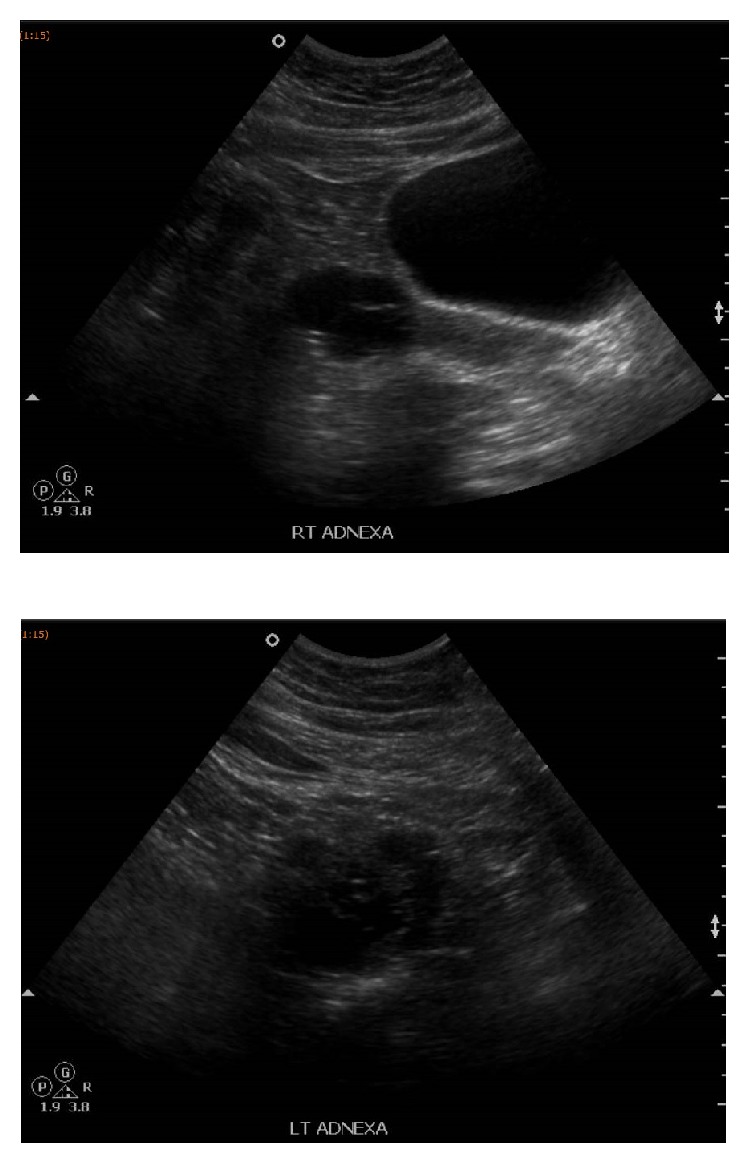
The ultrasound pelvis showed a multicystic avascular structure in the right adnexa measuring 4.5 x 4.3 x 3.3cm. Another multicystic avascular structure was seen in the left adnexa measuring 5.4 x 4.4 x 4.1cm. These could possibly represent polycystic ovaries containing multiple cysts.
